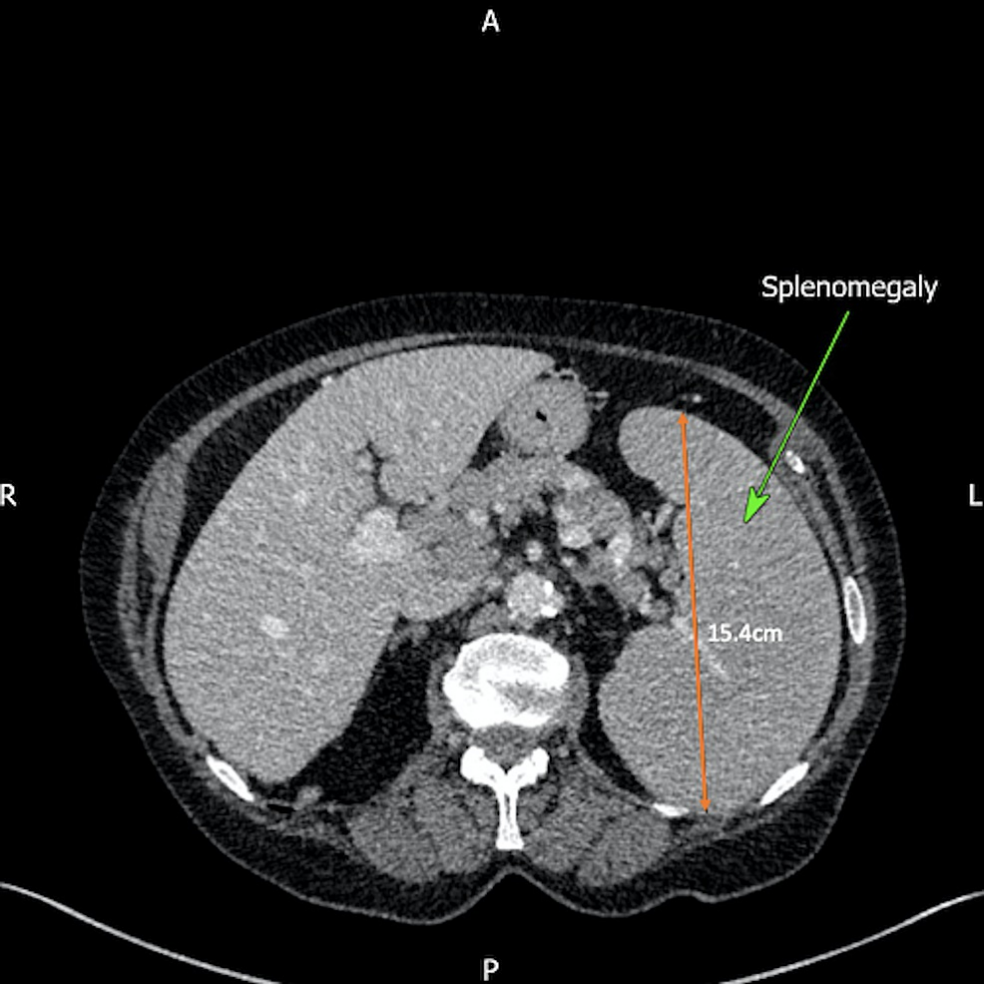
Computed tomography (CT) of the abdomen displaying evidence of splenomegaly (green arrow) with a measurement of 15.4 cm (orange arrow).
